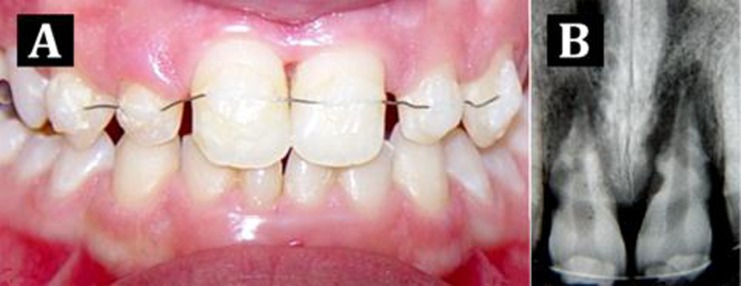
Initial clinical and radiographic aspects
